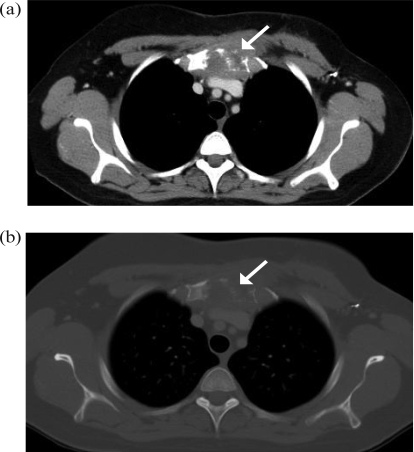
Computed tomography of the thorax, performed preoperatively, shows a soft-tissue mass with manubrial destruction (arrows). (A) Soft-tissue window. (B) Bone window.
